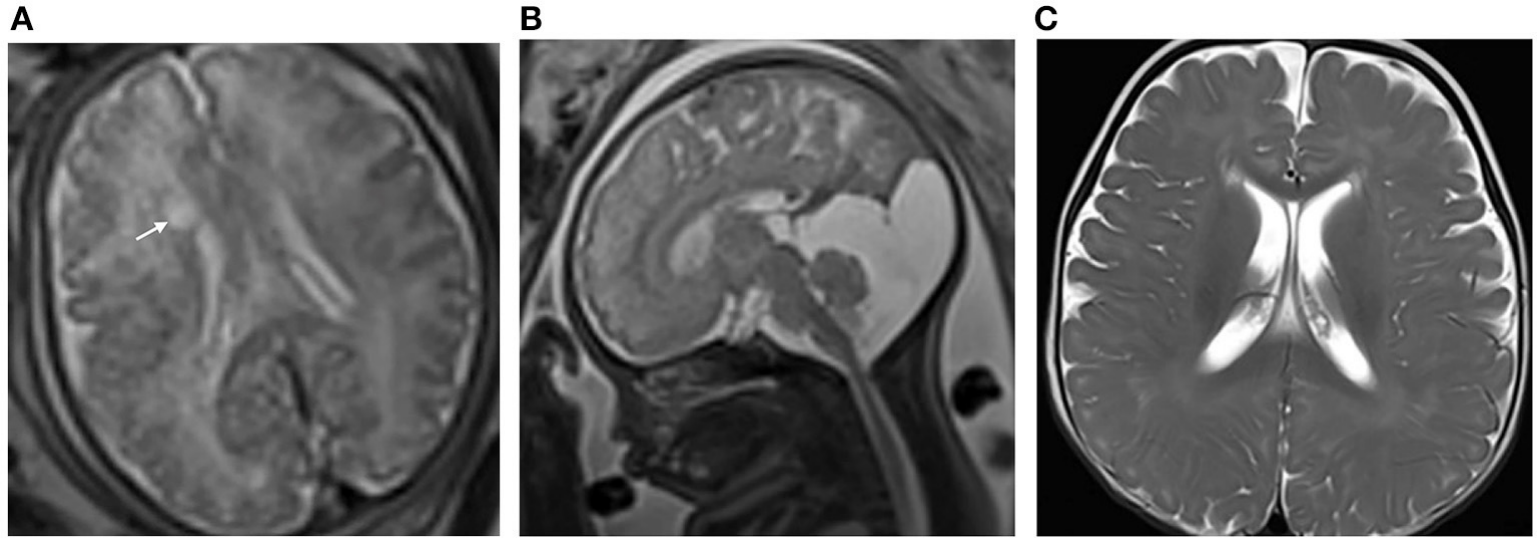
(A) Pregnant woman, 32 years old, with a fetus of 38 weeks' gestation. Fetal brain MRI shows a unilateral isolated periventricular pseudocyst (PVPC) located at the external angle of the lateral ventricle (white arrow); the anteroposterior diameter and height are 0.8 and 0.4 cm, respectively; (B) The additional finding is the large cyst in the cisterna magna (sagittal image); and (C) Female, 9 months after birth. The PVPC disappeared, and the Gesell Developmental Scale (GDS) test and developmental quotient showed a mild neurodevelopmental disability (DQ: Adaptive: 73, Gross Motor: 58, Fine Motor: 70, Language: 69, and Personal Social: 66).
